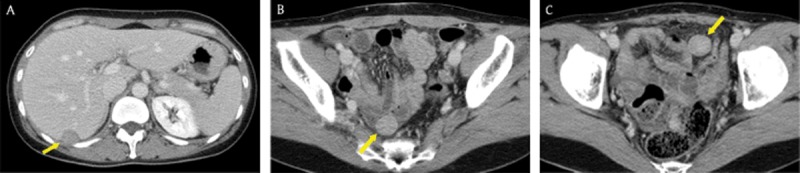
A 39-year-old woman whose surgical history included hysterectomy for uterine leiomyoma. Axial portal venous phase CT images showed multiple, well-circumscribed, solid masses (arrows) with mild-to-moderate enhancement in the peritoneal cavity. There were no abnormal lymph nodes, ascites, or omental haziness in the abdomen. The patient was diagnosed with leiomyomatosis peritonealis disseminata through percutaneous core needle biopsy under ultrasonography guidance.
